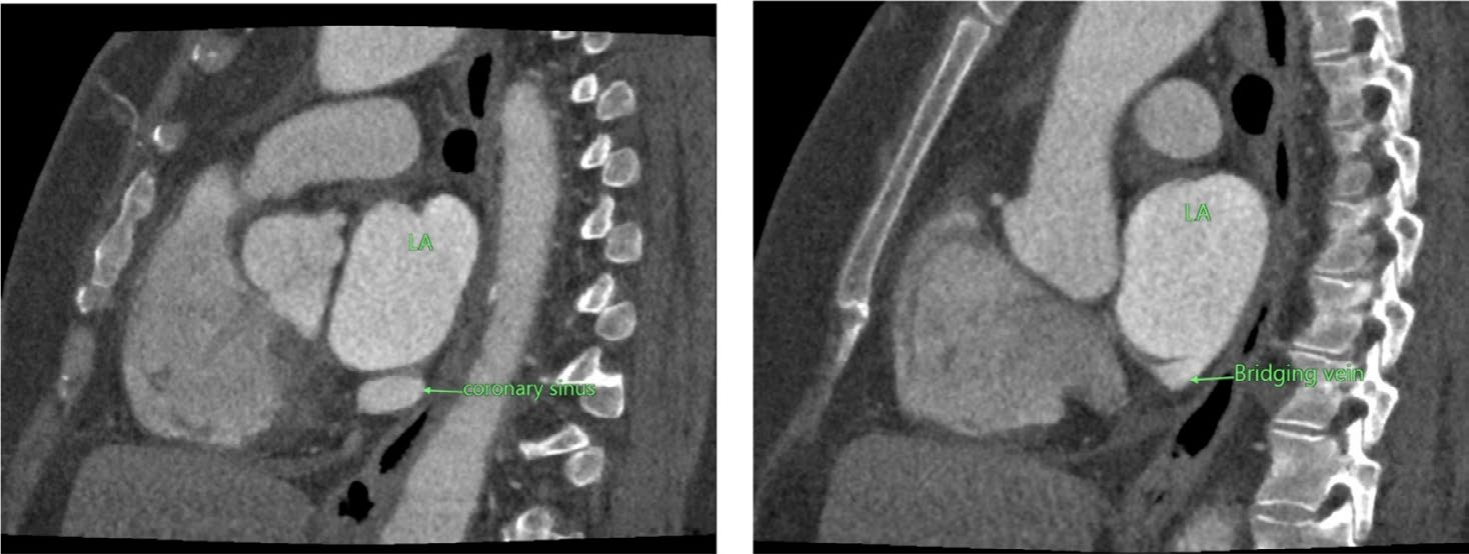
Computed tomography coronary angiogram sagittal views showing the indirect communication of the coronary sinus and left atrium through a bridging vein.
